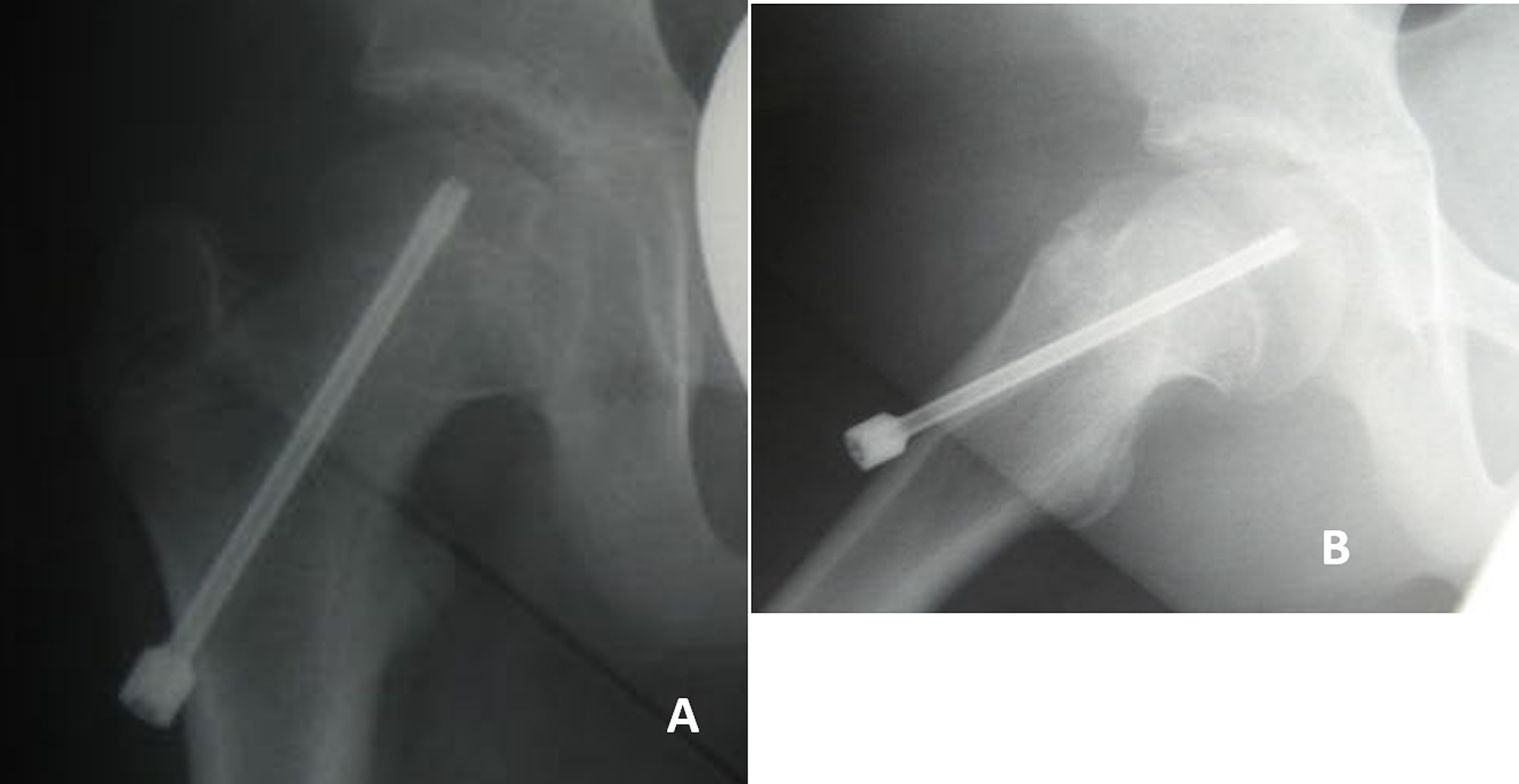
Postoperative imaging (3 years after first surgery). (A) Anteroposterior and (B) Rauenstein radiographs of the right hip, the lateral end of the screw was gradually incorporated into the femur and approached the cortex.
